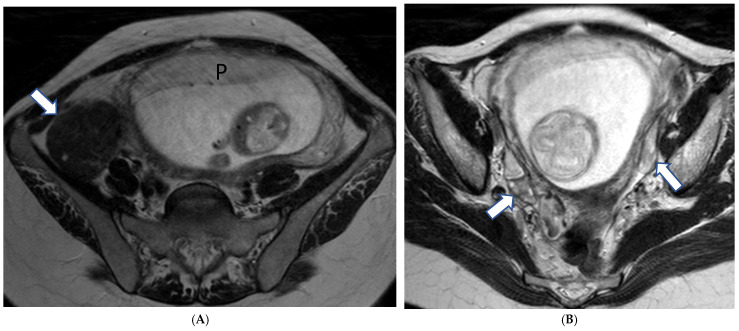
A 29-year-old pregnant woman at gestational week 21 with a suspicious right ovarian mass incidentally detected on routine second-trimester ultrasound: (A) Axial T2-weighted image shows a low signal intensity mass attached to the right side of the uterus typical of a pedunculated leiomyoma (arrow) (P: Placenta). (B) Axial T2-weighted image of the same patient at a lower level shows clearly the normal ovaries (arrows).
